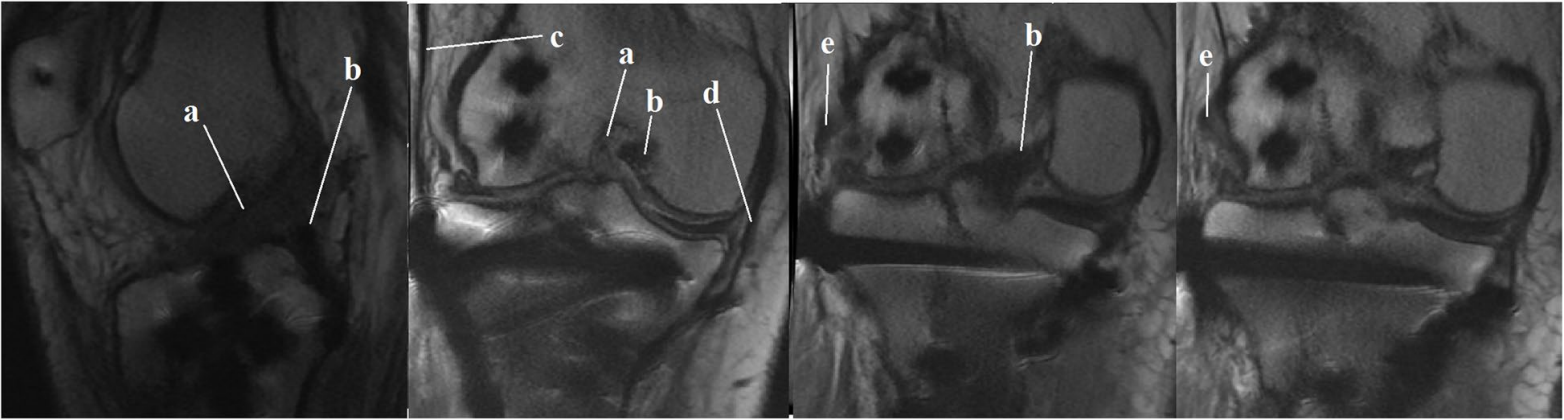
MRI at the latest follow up: a, anterior cruciate ligament; b,posterior cruciate ligament; c, tensor fascia lata; d, medial collateral ligament; e, fibular collateral ligament
